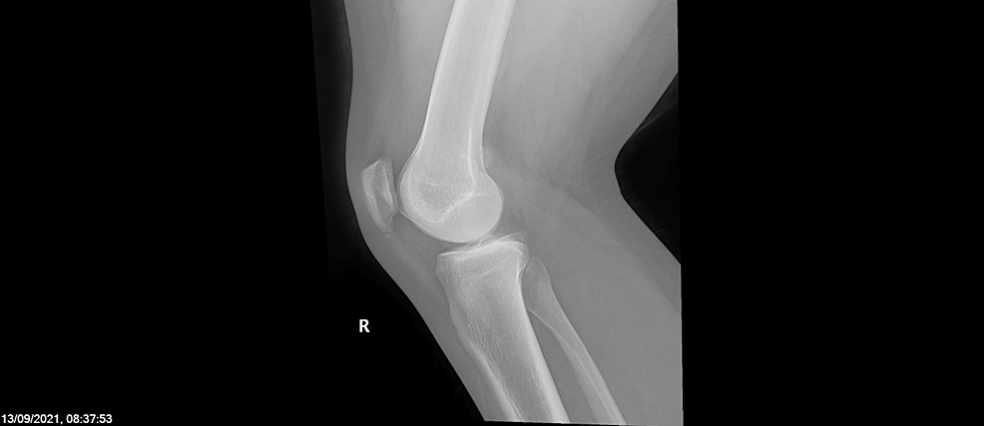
Lateral right knee plain radiograph
